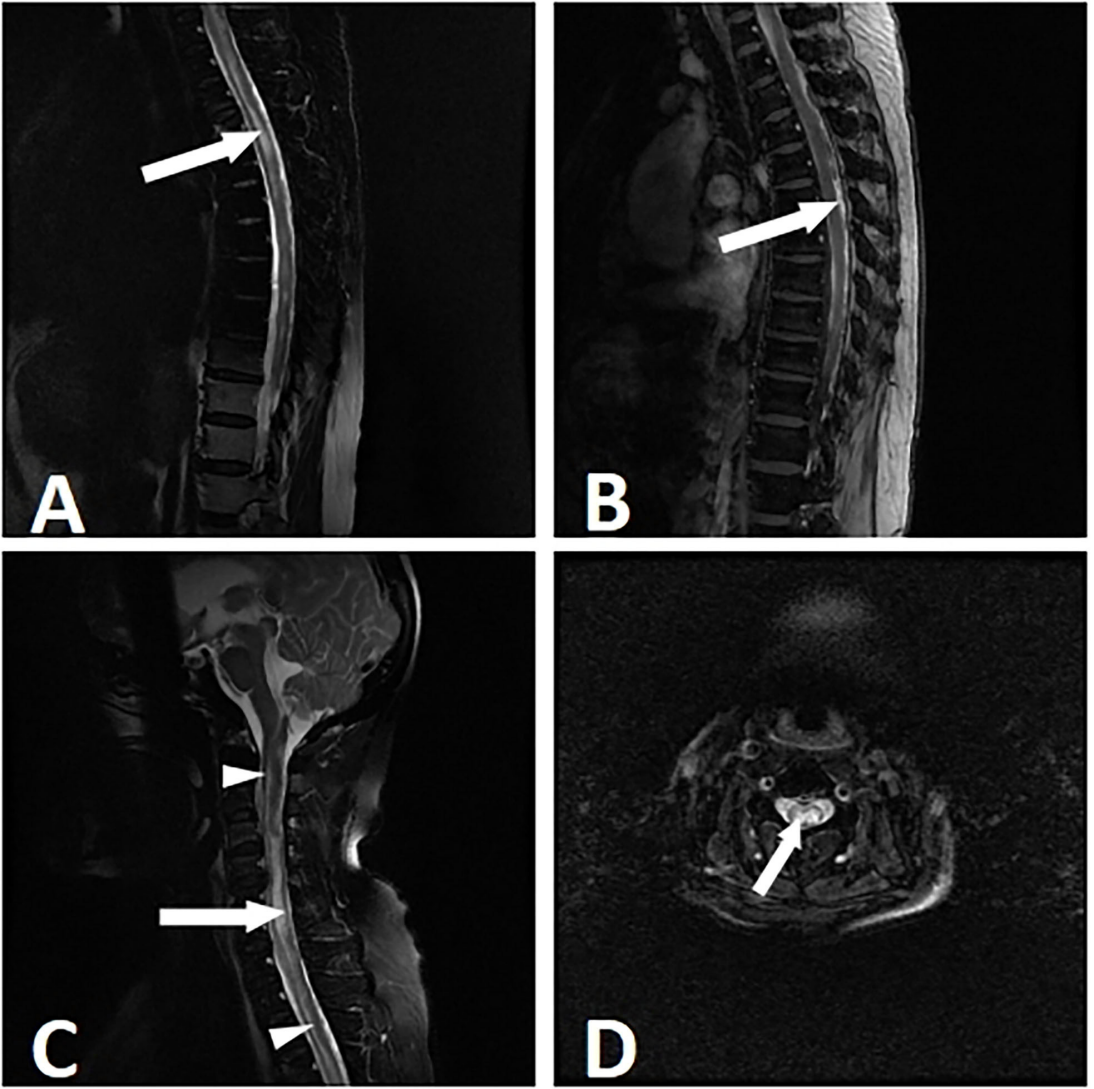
Myelitis in a 53-year-old woman with tuberculous meningitis. The patient suffered from paraparesis with the duration of 8 weeks. MRI showed longitudinally myelitis. T2-weighted imaging showed a stripped-like hyperintensity (A). In the T1 contrast-enhanced sequence, uneven enhancements of the lesions were noticed (B). T2-weighted sagittal and axial images showed an uneven enlargement of the spinal cord and syringomyelia. The syringomyelia manifested as a stripe of intramedullary near-water signals (long arrow) and enlargement of the central canal (short arrow) (C,D).
